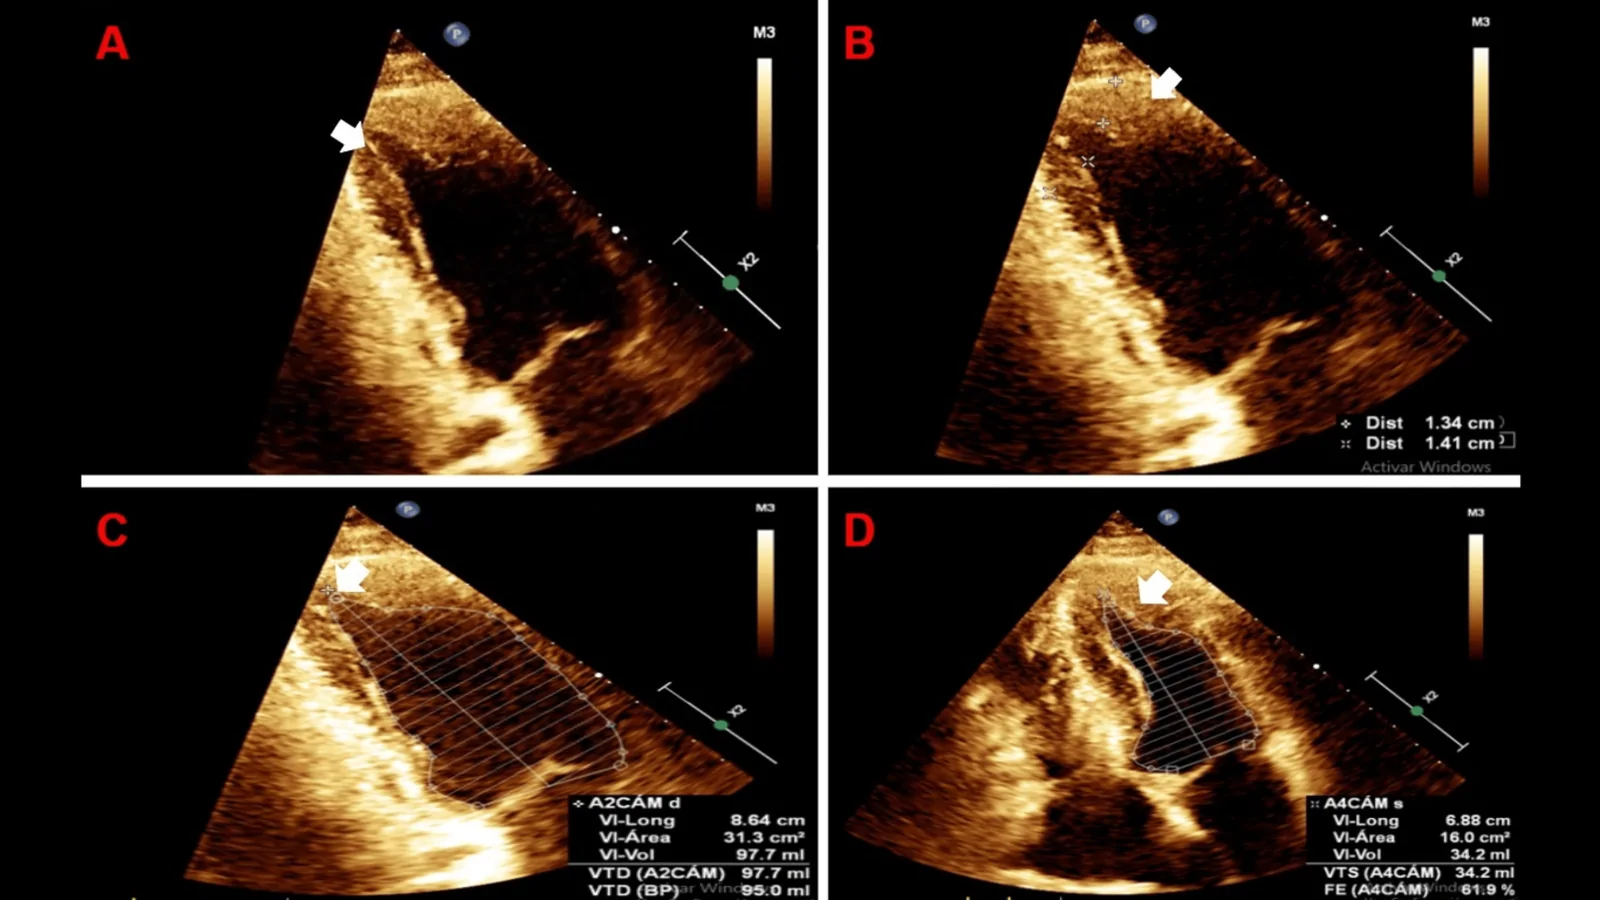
Transthoracic echocardiography Transthoracic echocardiography demonstrating focal hypertrophy confined to the left ventricular apex (A, white arrow), with a maximal apical wall thickness of 14 mm (B, white arrow). Apical four-chamber views reveal the characteristic spade-shaped left ventricular cavity ("ace-of-spades" appearance) during end-diastole (C and D, white arrows), a hallmark of apical hypertrophic cardiomyopathy (Yamaguchi syndrome).
